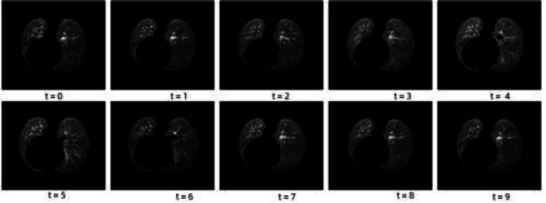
Examples of 4D CT images of the lung region.
